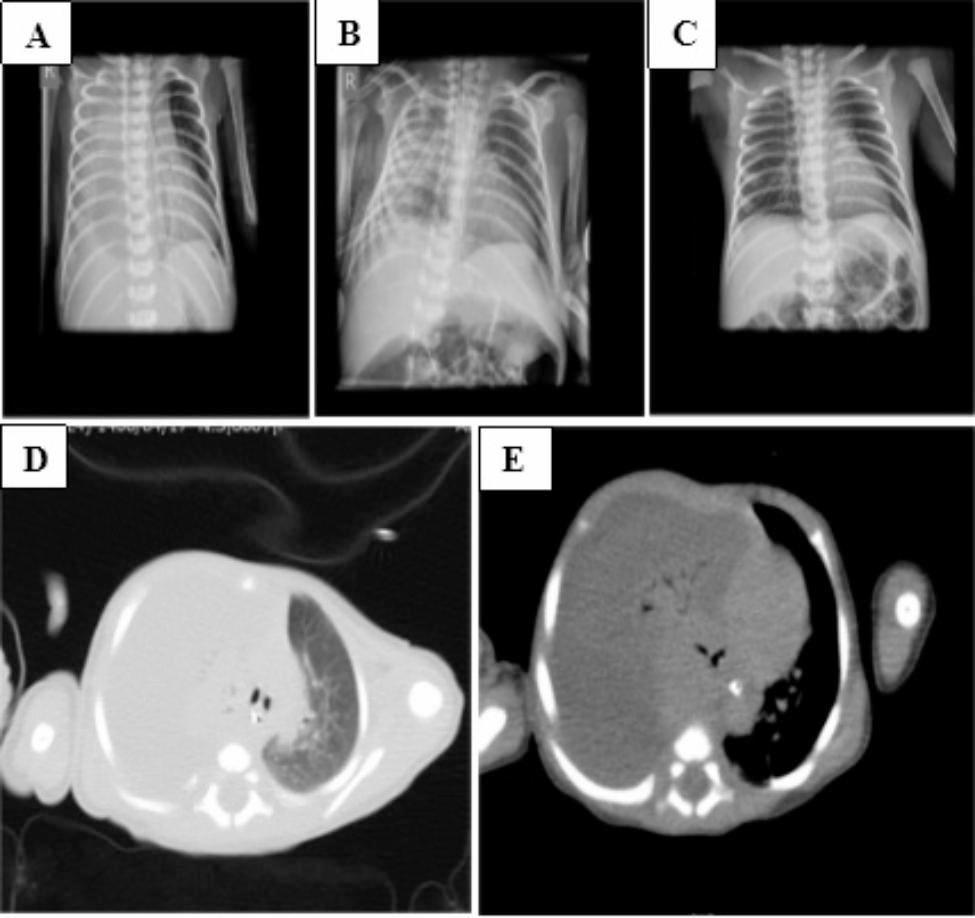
Chest radiographs of patient before (A) and after (B) chest tube insertion. Chest radiograph at the end of treatment (C). Chest CT-Scan showing a large volume of empyema on the right side (D-E).
